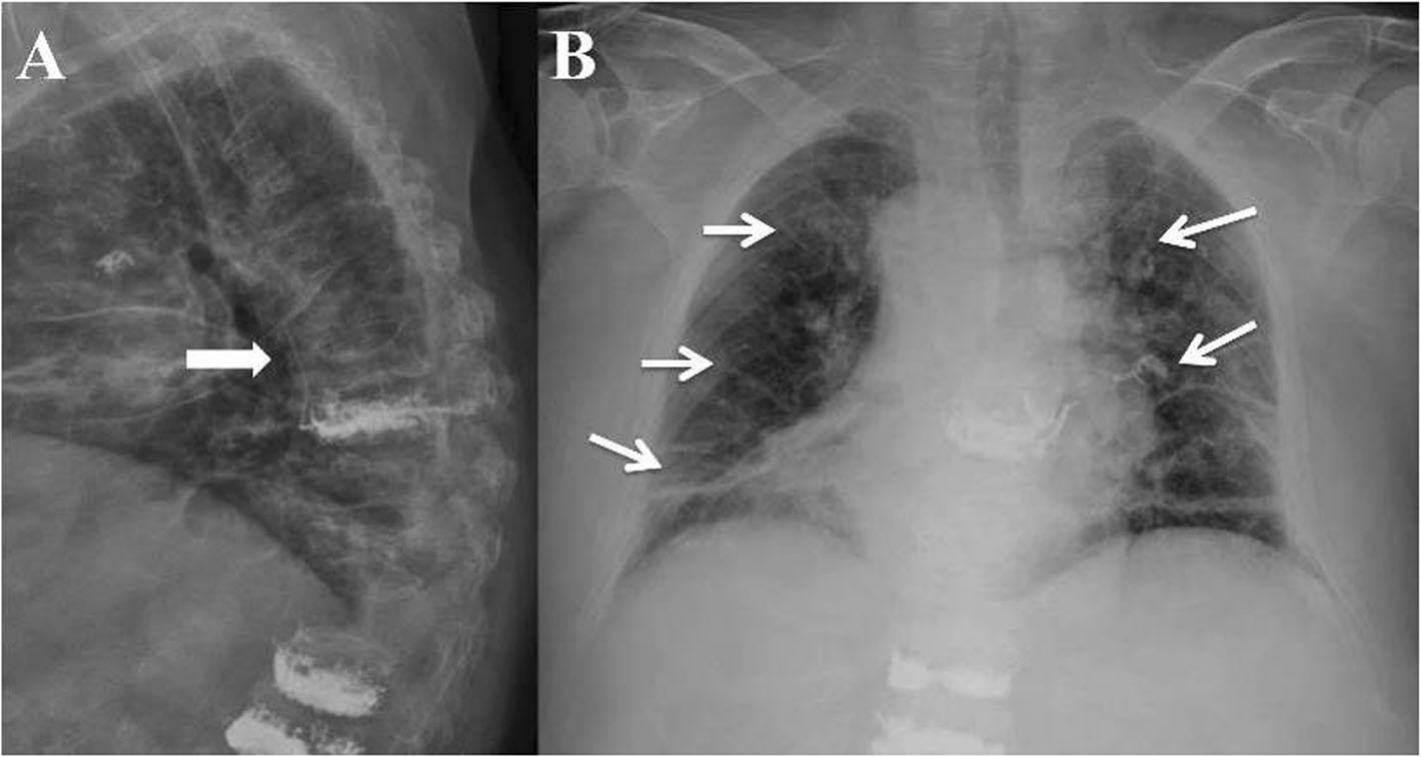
Postoperative lateral spinal radiograph 1 day after PVP with PMMA of osteoporotic fractures at levels T8, T12, and L1 in an 86-year-old male. The patient had dyspnea and chest pain after operation; type S leakage along the paravertebral veins (arrow) was noted (a). High-density PMMA cement with a tubular shape and branching opacities (arrows) distributed throughout the lungs and corresponding to lung vessels, caused pulmonary embolism, as seen on chest radiograph (b). After oxygen support and anticoagulation therapy for 3 days, follow-up revealed no symptom aggravation
